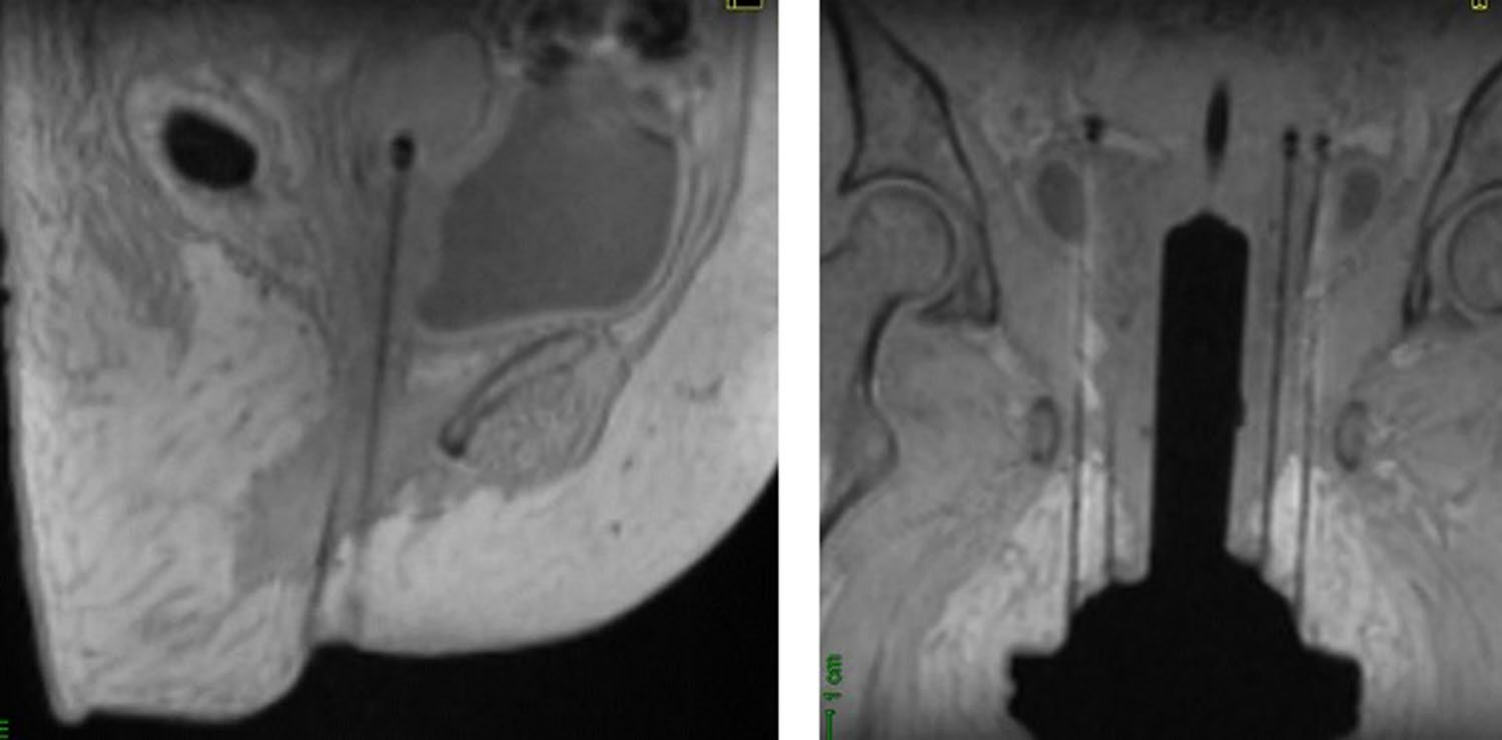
MRI images showing a “ballooning” artifact at the end of the titanium needle
